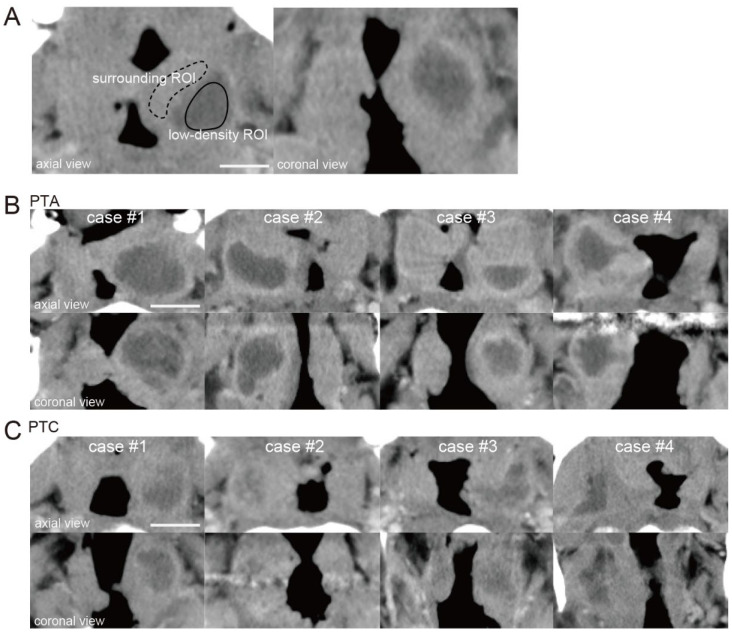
CT images of cases of peritonsillar abscess (PTA) and peritonsillar cellulitis (PTC). (A) ROI setting. The ROI was selected from the low-density area identified on the axial contrast-enhanced CT image (low-density ROI, solid circle). The surrounding area of the low-density area was set as the surrounding ROI (surrounding ROI, dotted circle). ROI, region of interest; scale bar, 20 mm. (B) Representative CT images of PTA cases (case #1 to #4). The upper images show axial sections, and the lower images show coronal sections. Scale bar, 20 mm. (C) Representative CT images of PTC cases (case #1 to #4). Scale bar, 20 mm.
